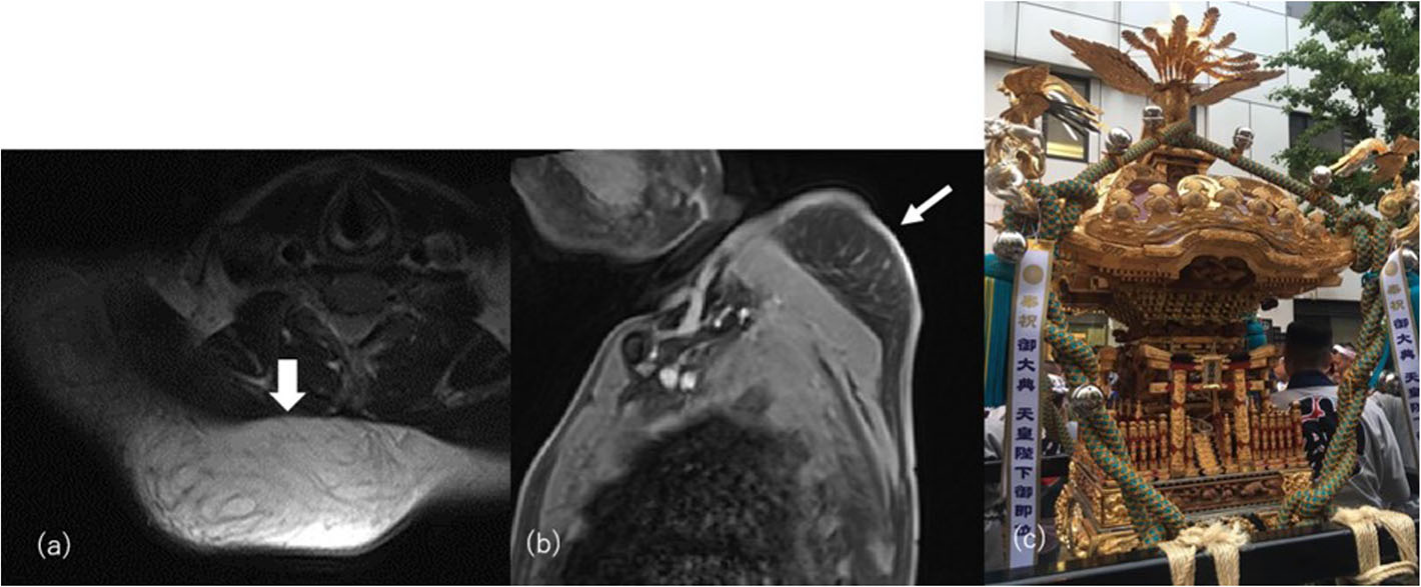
A 60-year-old man with post-traumatic pseudolipoma on the right posterior neck. He had a history of carrying a mikoshi (Japanese portable shrine) daily. a Axial T1-weighted and b sagittal post-contrast fat-sat T1-weighted images show non-capsulated subcutaneous fat tissue proliferation on the right posterior neck (arrows). c Photograph of a mikoshi
